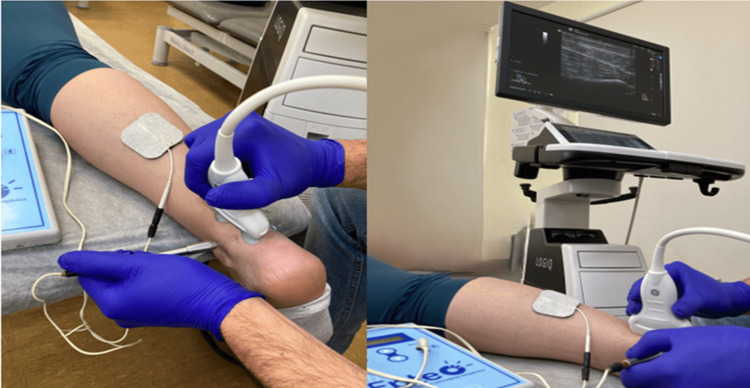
Ultrasound-guided percutaneous electrolysis technique.
